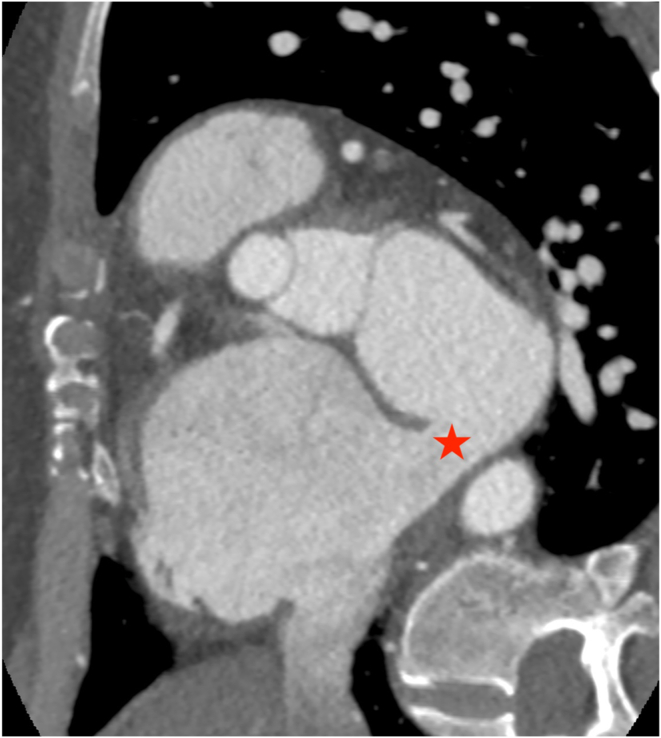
Cardiac computed tomography, oblique sagittal plane, cardiac basal-LV short-axis view demonstrates a completely unroofed CS with an unrestricted LA-CS communication *(star)*.
